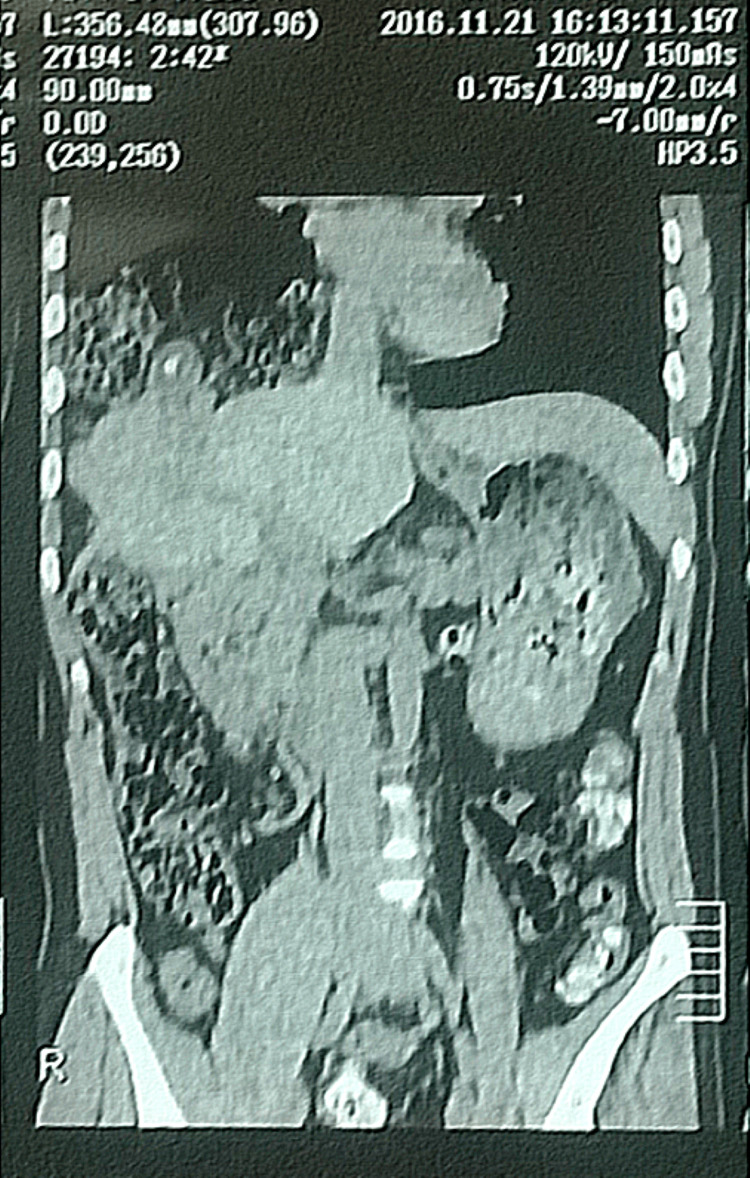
CT scan image showing herniated contents in the right hemithorax with a visible calculus in the gallbladder - Coronal view CT - Computed Tomography
